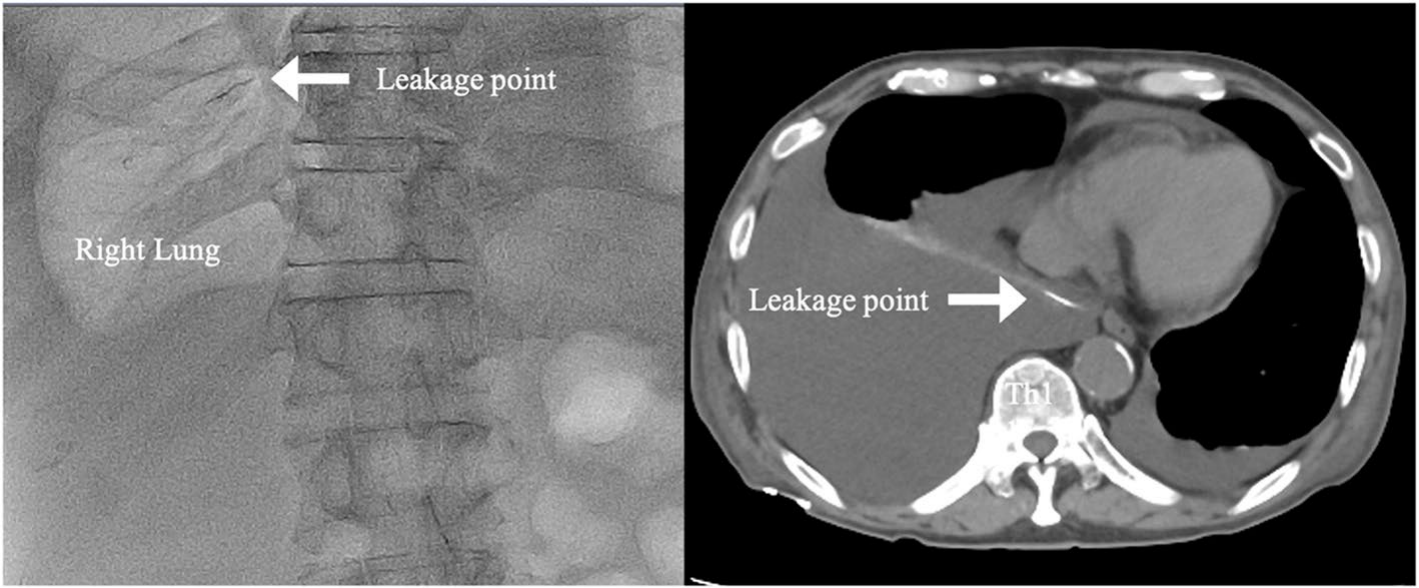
Intranodal lymphangiography showing leakage of Lipiodol from the thoracic duct into the right thoracic cavity. With the patient in the supine position, an ultrasound-guided right-sided inguinal lymph node injection of 11-ml Lipiodol was performed to follow the flow of contrast by fluoroscopy (Fig. 3). Approximately 20 min later, the injection of Lipiodol into the lymphatic vessel was confirmed under fluoroscopy. Immediately, CT was taken, which showed leakage of Lipiodol from the thoracic duct at the level of Th 11–12
